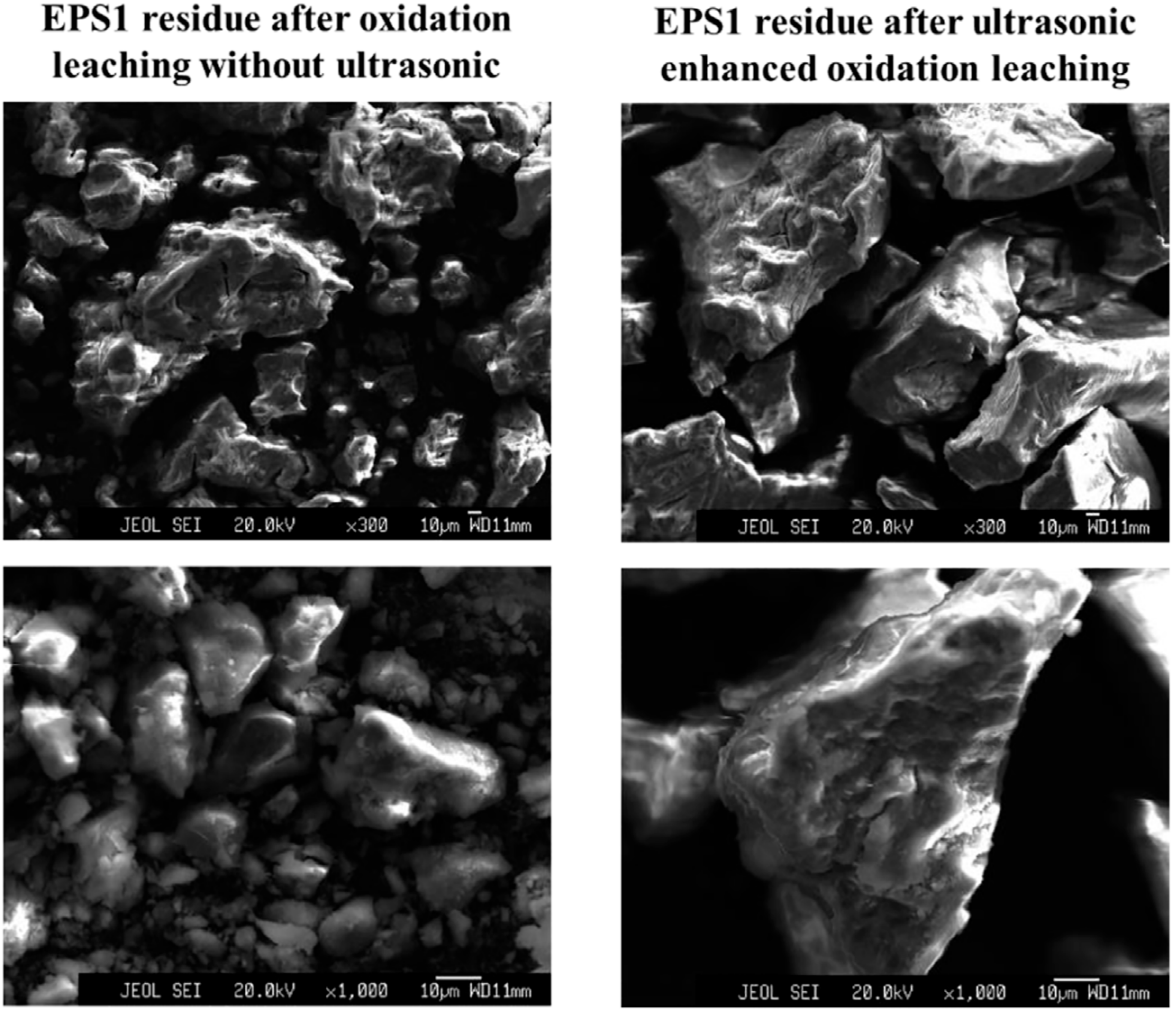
Particle morphology of chromium sludge (EPS1) after oxidation leaching with and without ultrasonic treatment.
